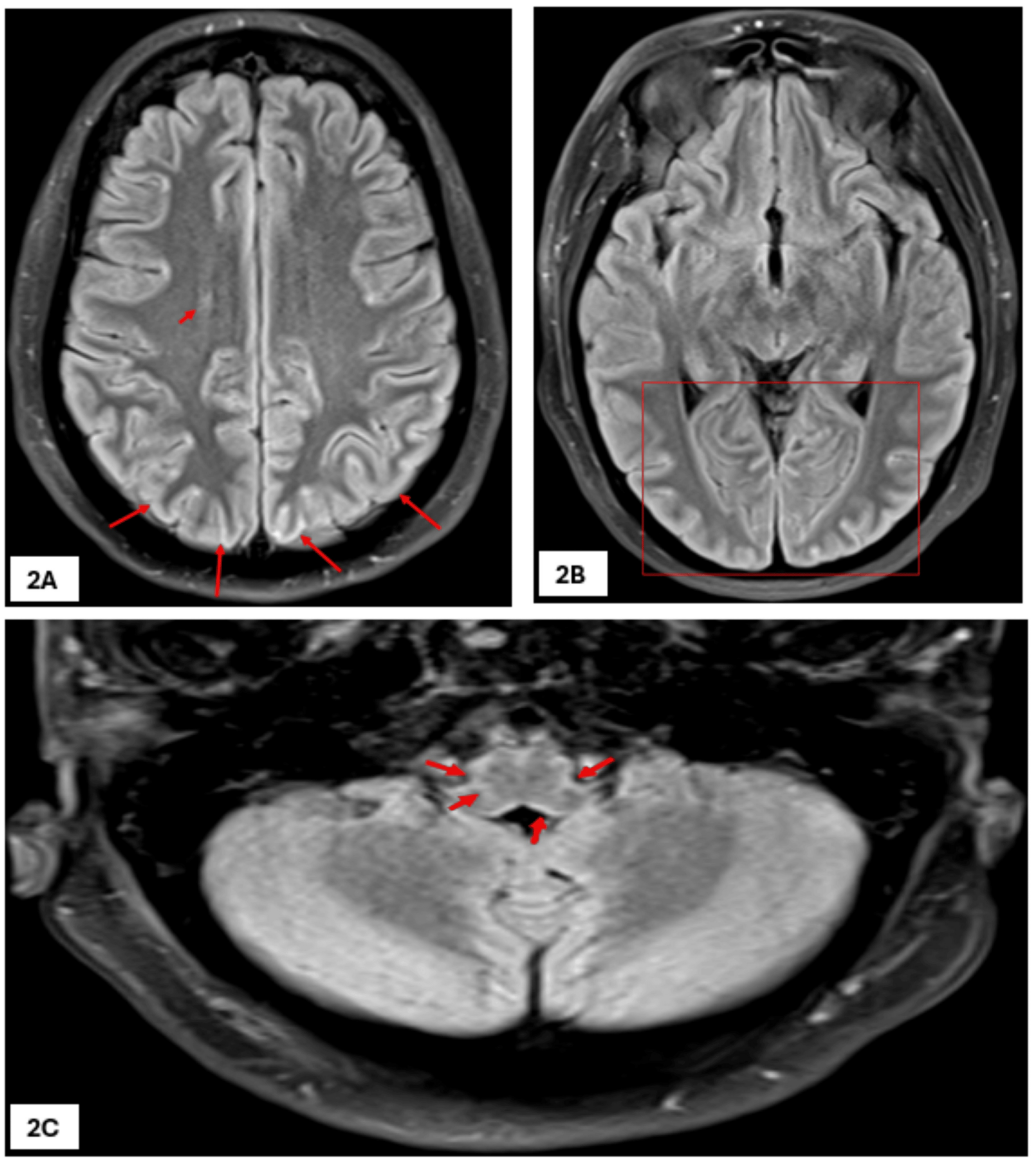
First MRI scan of brain (FLAIR images). Two-dimensional axial fluid-attenuated inversion recovery (FLAIR) images showing right frontal deep periventricular focus of FLAIR signal elevation (short arrow in 2A) with subtle cortical swelling and elevated FLAIR signal seen along high parietal (long arrows in 2A), posterior temporal and occipital polar regions (rectangle in 2B), and medulla oblongata (short arrows in 2C).
